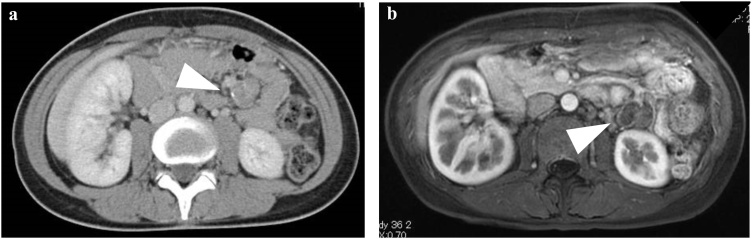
Enhanced CT scan shows a heterogeneously enhancing mass. The latter had mottled calcifications and a cystic portion. It measured 38 mm in length in abdominal cavity (a). T2-weighted MRI showed a low intensity mass separate from the bowel (b).
